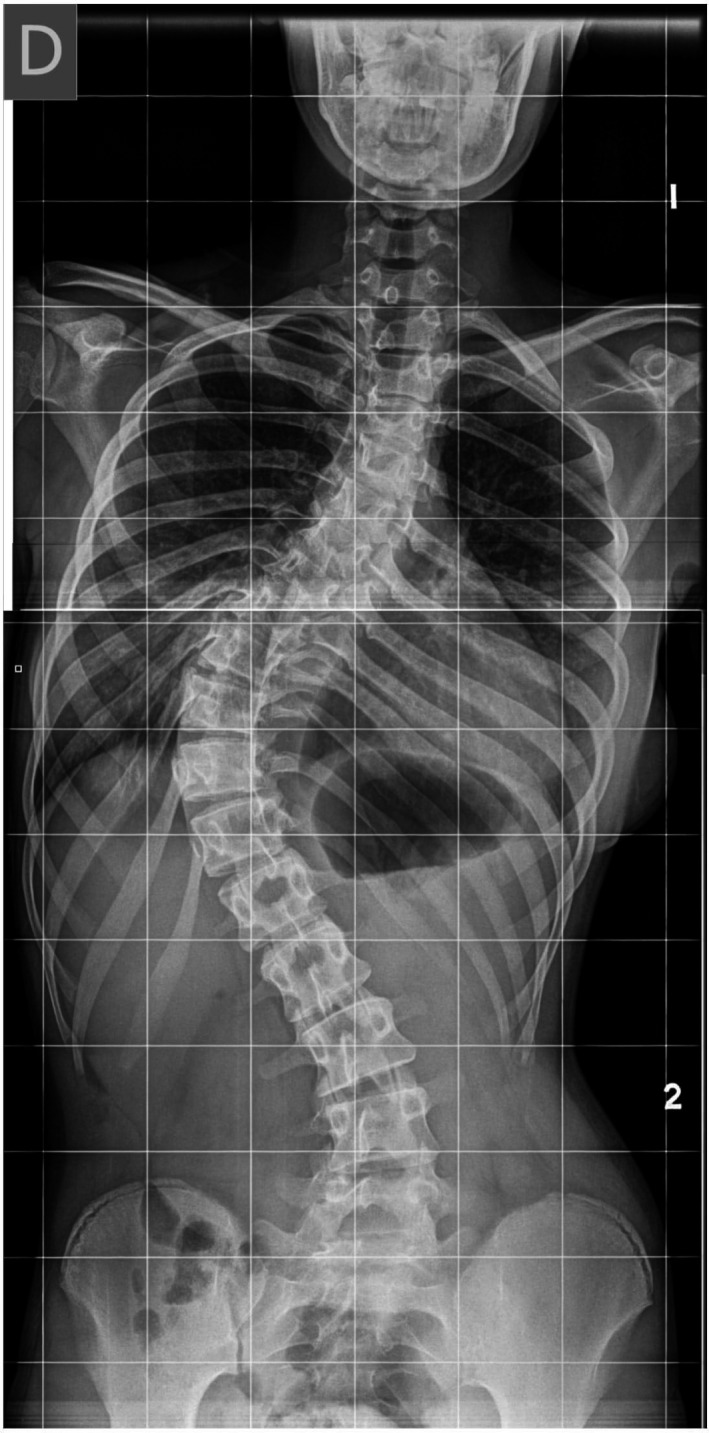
Radiography showing the dorso‐lumbar scoliosis, before correction
